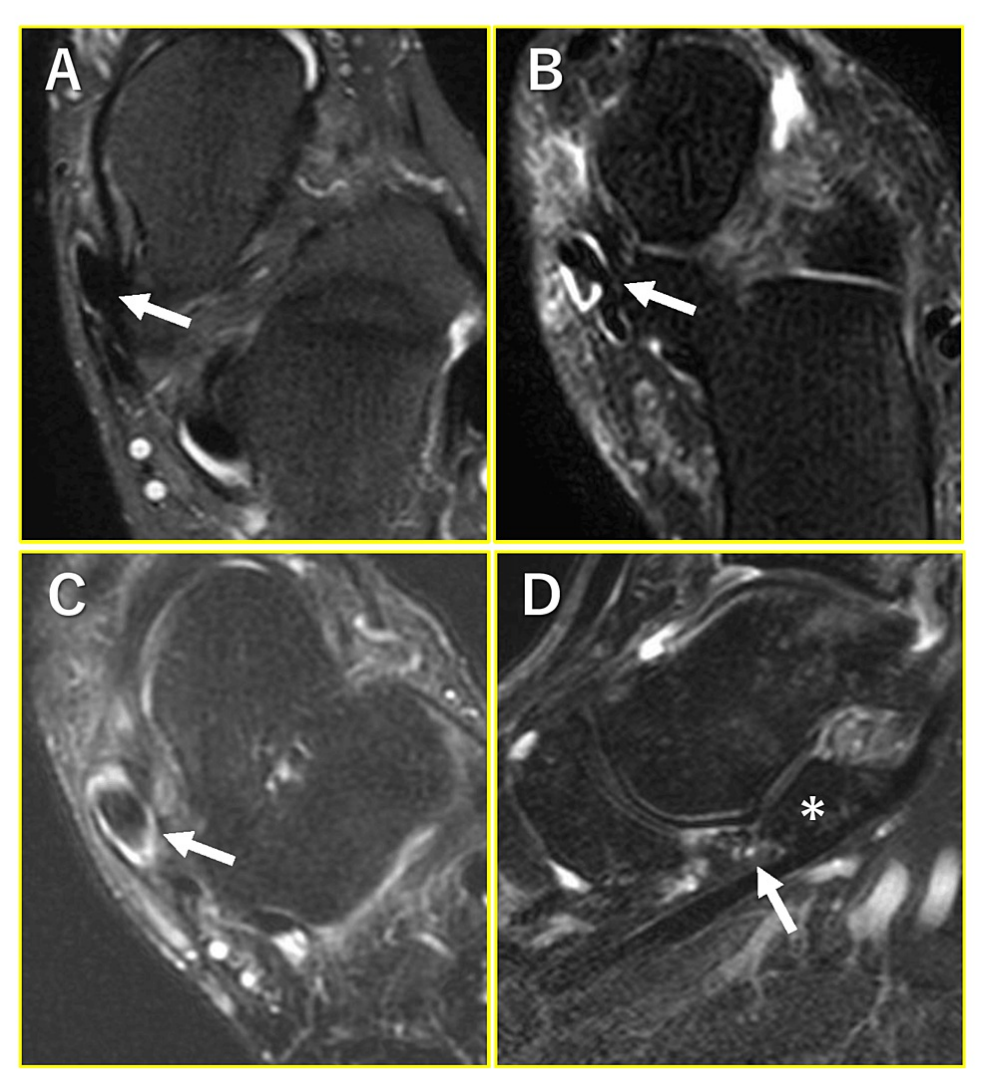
Representative MRI images of the posterior tibial tendon (PTT) and spring ligament. (A) Normal PTT. (B) Paratendonitis of PTT. (C) Tendonitis of PTT. The the arrows indicate PTT. (D) Abnormal finding of the spring ligament. The arrow indicates the spring ligament and the asterisk (*) indicates sustentaculum tali.
